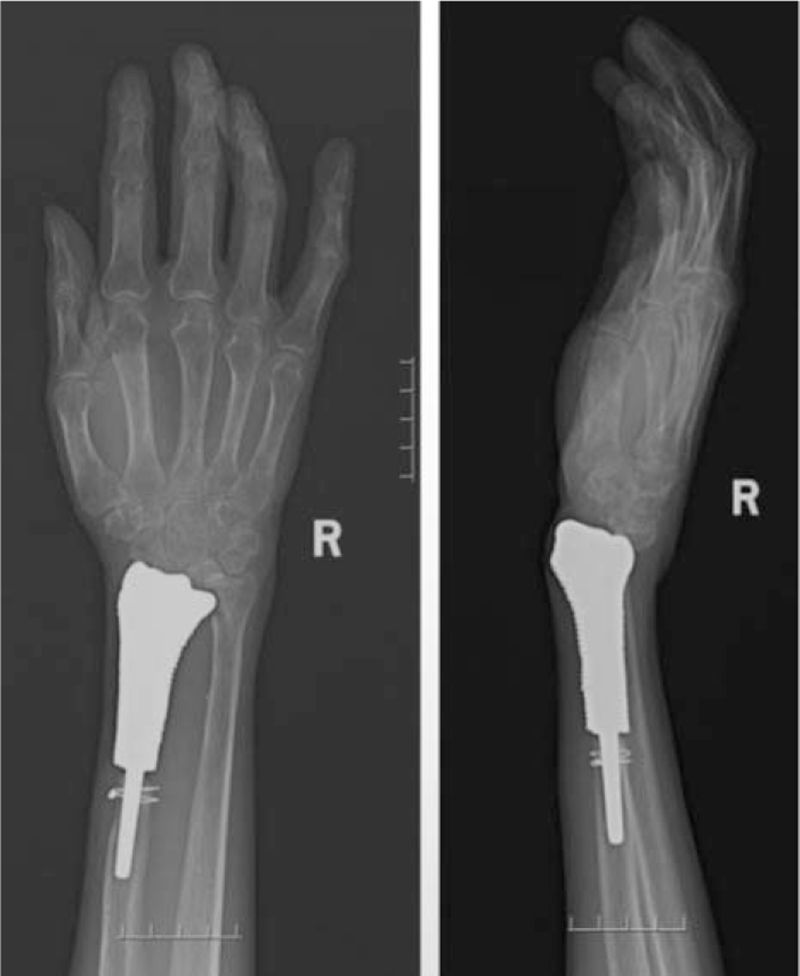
X-ray films 2 year after operation: There was no failure or loosening of implant.
